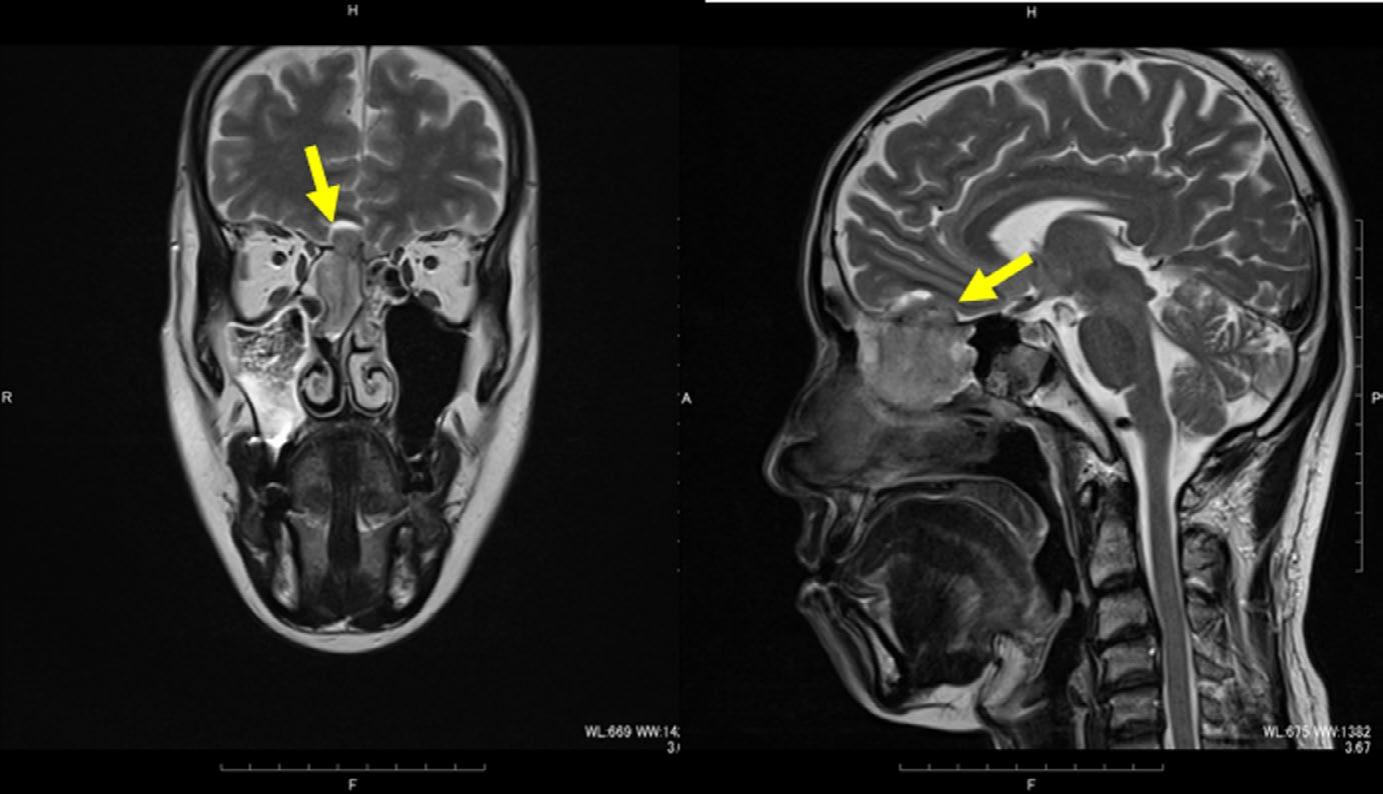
MRI image after neoadjuvant chemotherapy still showing intracranial invasion of the tumor to the brain and the most anterior aspect of the sphenoid sinus
